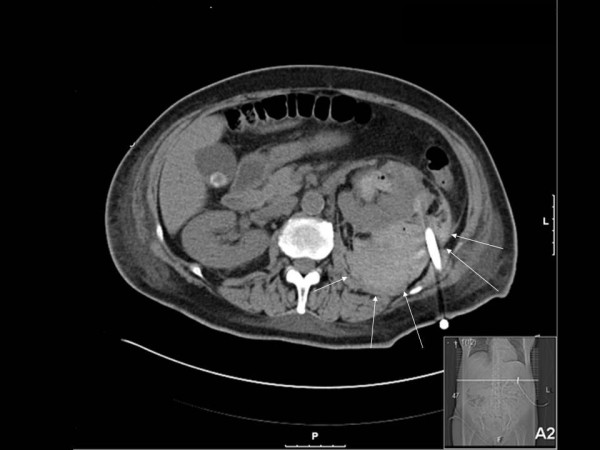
Abdominal computed tomography scan prior to angiography. A large left perinephric haematoma with active extravasation of contrast is identified (arrows).
